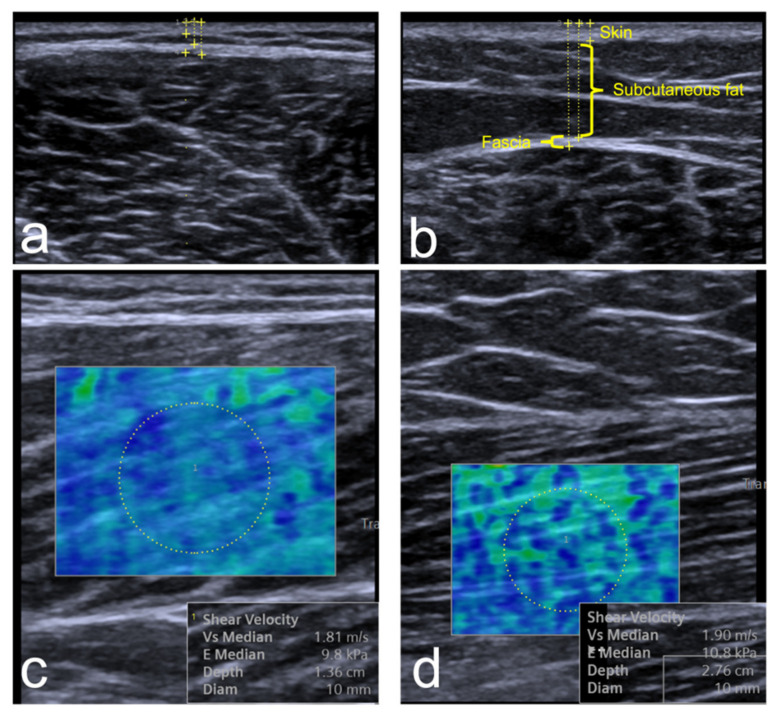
A sample of the elastography and ultrasound thickness measurements for the vastus lateralis (a,c) and biceps femoris (b,d). The thickness measurements show the calibers placement for skin, subcutaneous fat, and fascia. The SWE images show the hue color distribution of the stiffness and the Young’s modulus kPa results.
